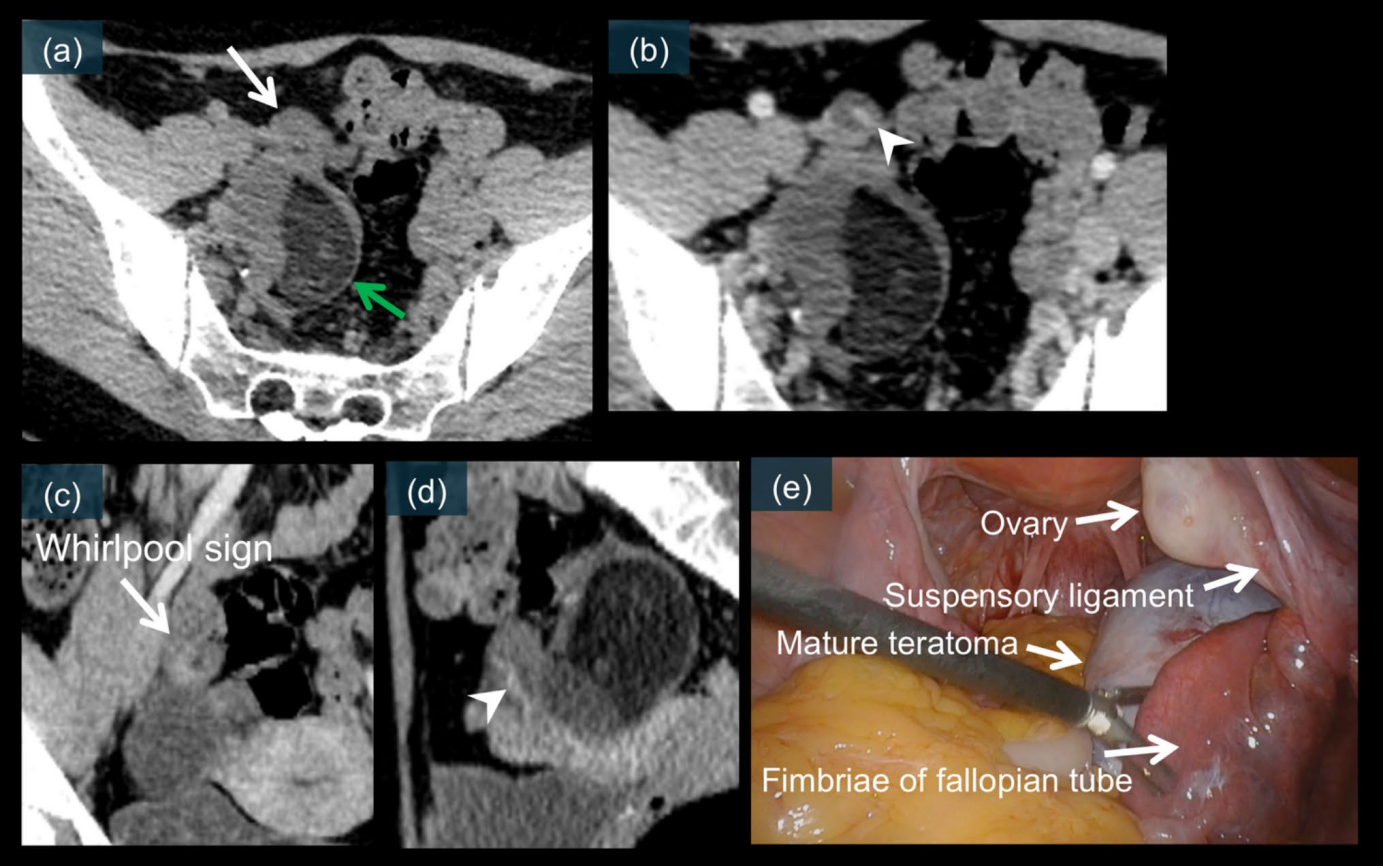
A 35-year-old female. a is an unenhanced CT image. b–d are contrast-enhanced CT images. The mature cystic teratoma is located in the midline of the pelvis. On unenhanced CT, a mass-like structure (white arrow) is observed adjacent to the mature cystic teratoma (green arrow) (a). On contrast-enhanced CT, particularly in the coronal plane, a whirlpool sign is clearly visualized (c, white arrow). The whirlpool sign is not clearly visible in the axial and sagittal planes (b and d). There is an elongated enhancement within the structure, suspected to be the ovarian artery (b and d, white arrowhead). e is a laparoscopic image, which reveals a 360° torsion of the mature cystic teratoma of the right fallopian tube. Furthermore, the ovarian ligament and ovary are not twisted
